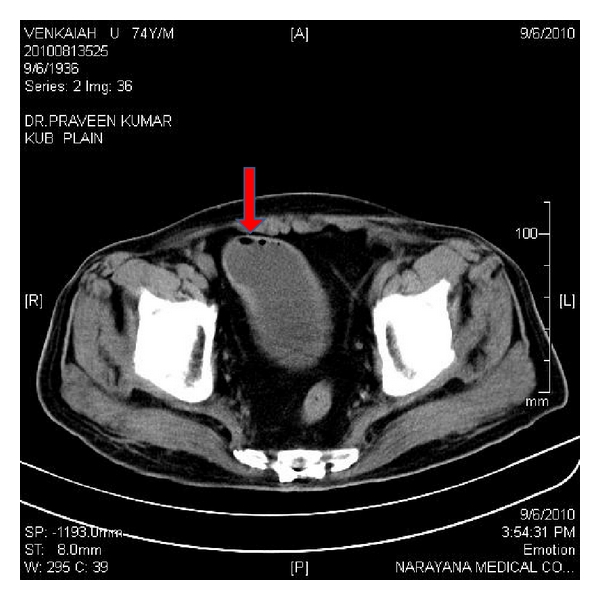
CT KUB showing emphysematous cystitis.
